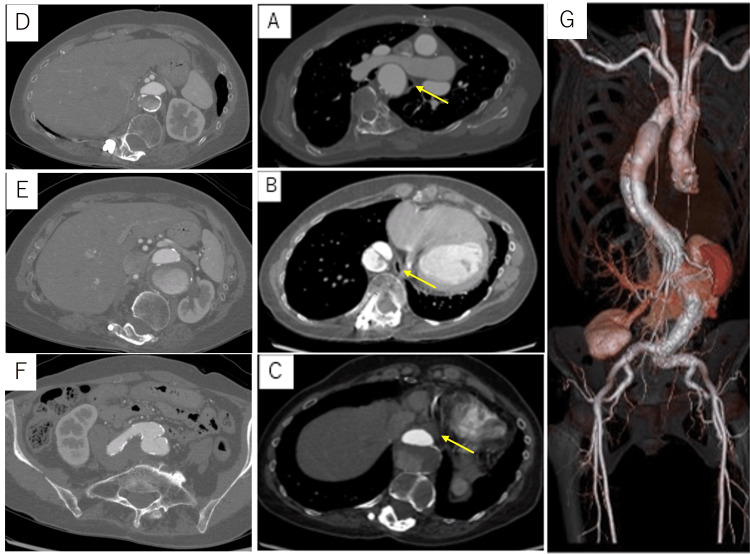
Patient’s postoperative CT findings The tortuous descending aorta was located just behind the esophagus at the second thoracic vertebrae level (A), which ran along the right side of the spinal column to the first lumbar vertebra (B), and then returned to the usual position (C). Abdominal vessels bifurcated from the true lumen (D). Dilatation of the aorta was observed from the second thoracic vertebral level with a maximum diameter of 55 mm at the ninth vertebral level (E). False lumen continues to bilateral common iliac bifurcation (F). (G) Three-dimensional CT image of the thoracoabdominal aneurysm. The arrows indicate the location of the esophagus.
